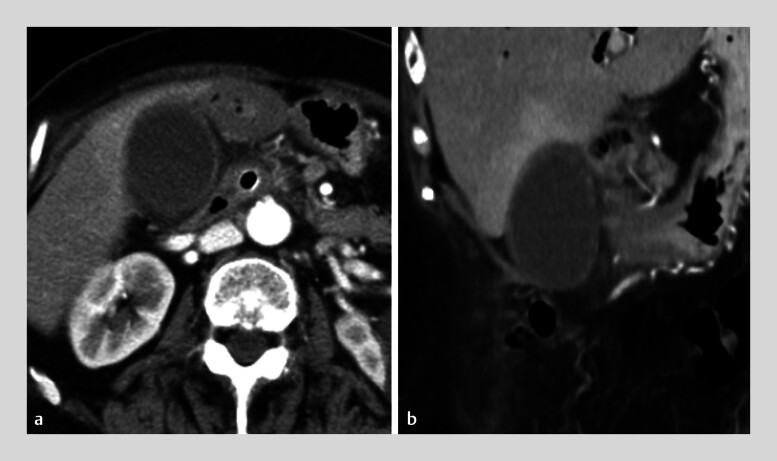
Contrast-enhanced computed tomography scans showing gallbladder enlargement.
